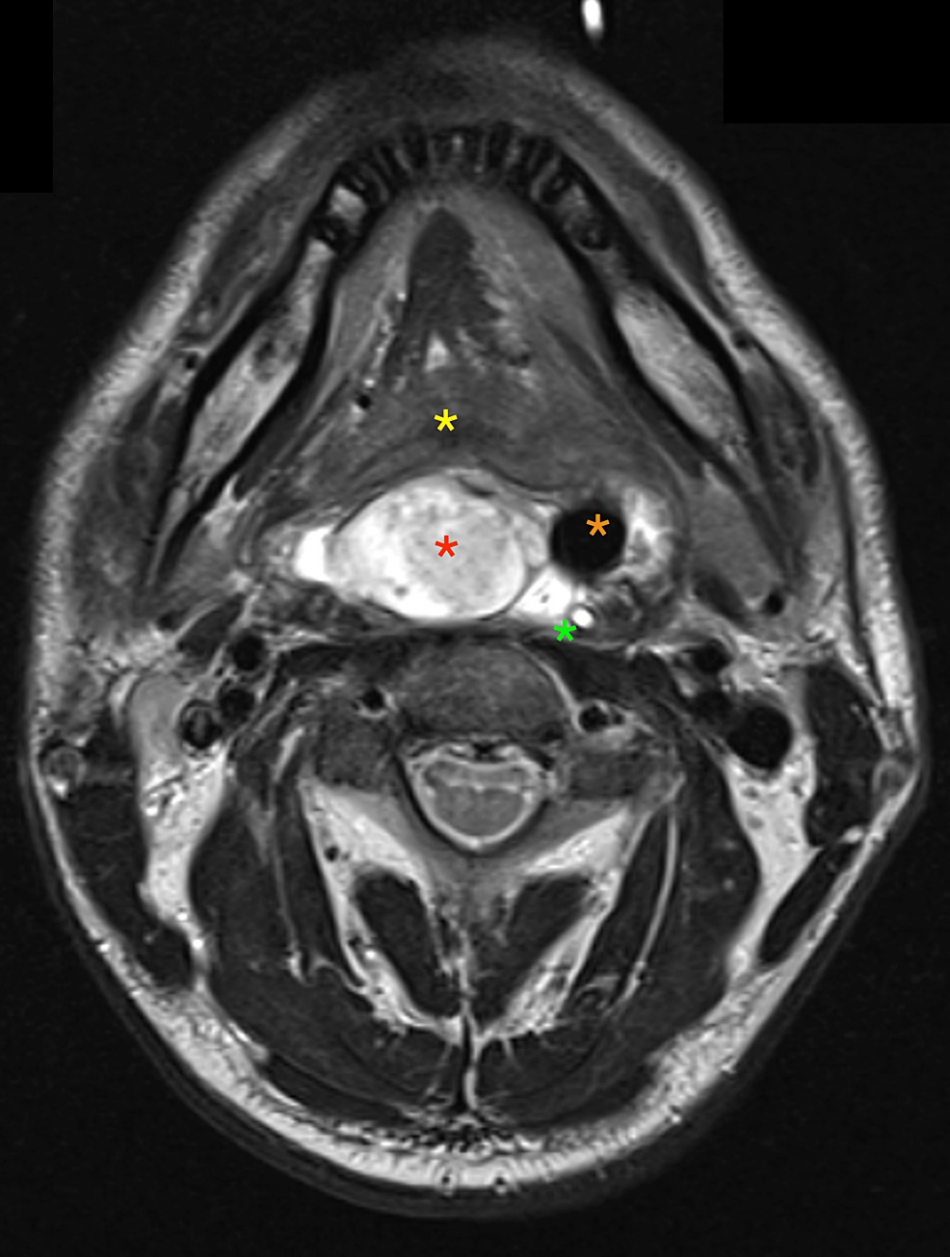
T2-weighted MRI scan of the neck with axial cut showing a large laryngeal mass (red asterisk) occupying the laryngeal inlet and compressing the base of the tongue. The yellow asterisk shows the tongue, the orange asterisk shows the upper airway partially compressed by the mass, and the green asterisk shows the nasogastric tube.
